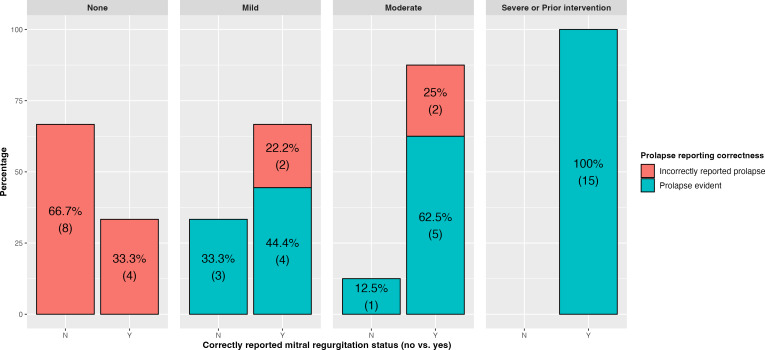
Accuracy of reporting mitral valve prolapse and mitral regurgitation (MR), stratified by MR severity on baseline echocardiogram. Each panel represents a different grade of MR and is split into 2 bars indicating correct MR reporting (blue) and incorrect MR reporting (red).
